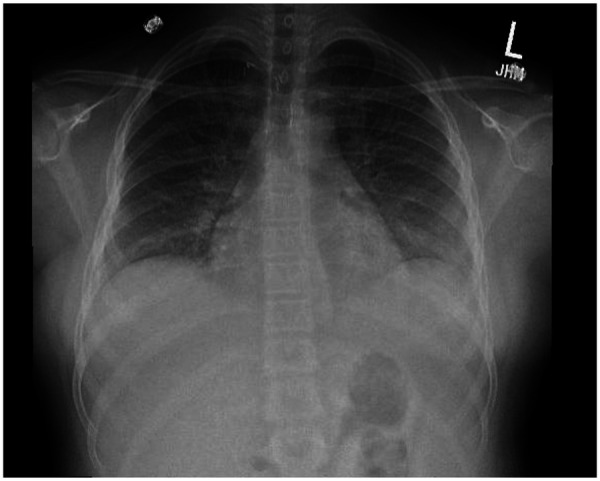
Chest xray PA view demonstrating bilateral pleural effusions and trace pericardial effusion at presentation.
